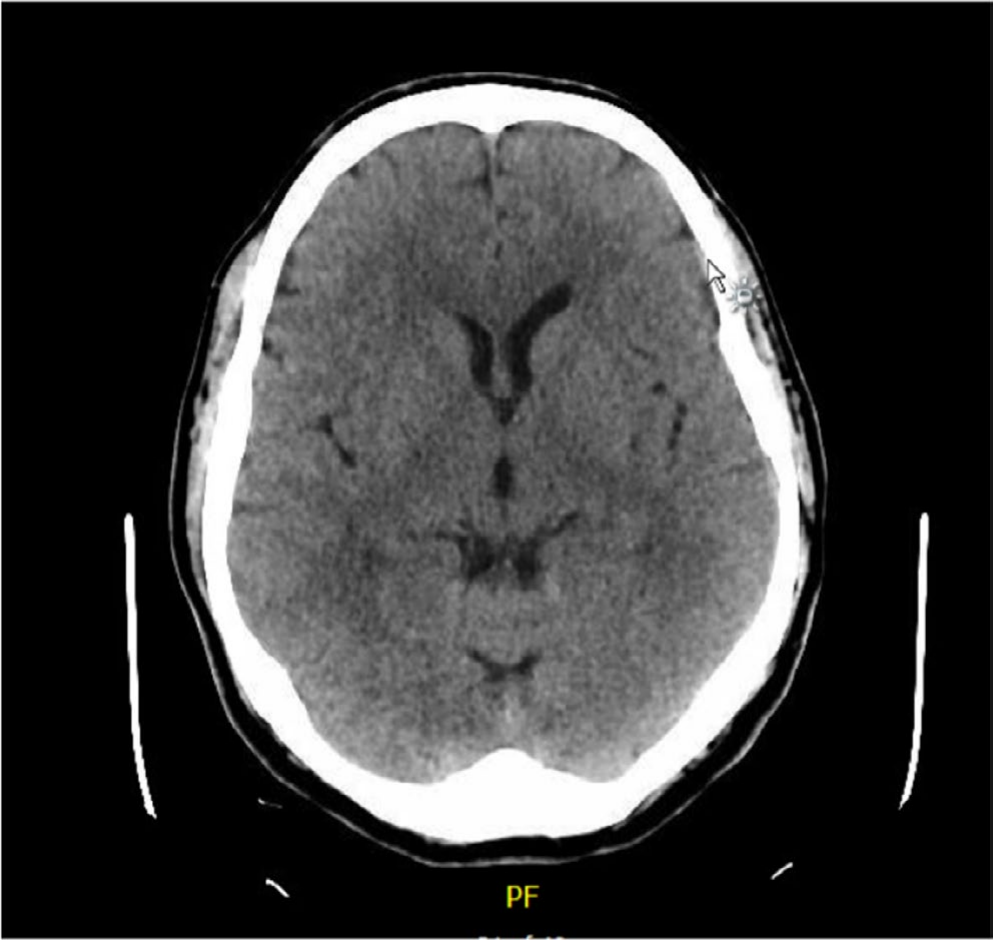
Transverse slice of initial CT Head on first presentation. Normal scan
